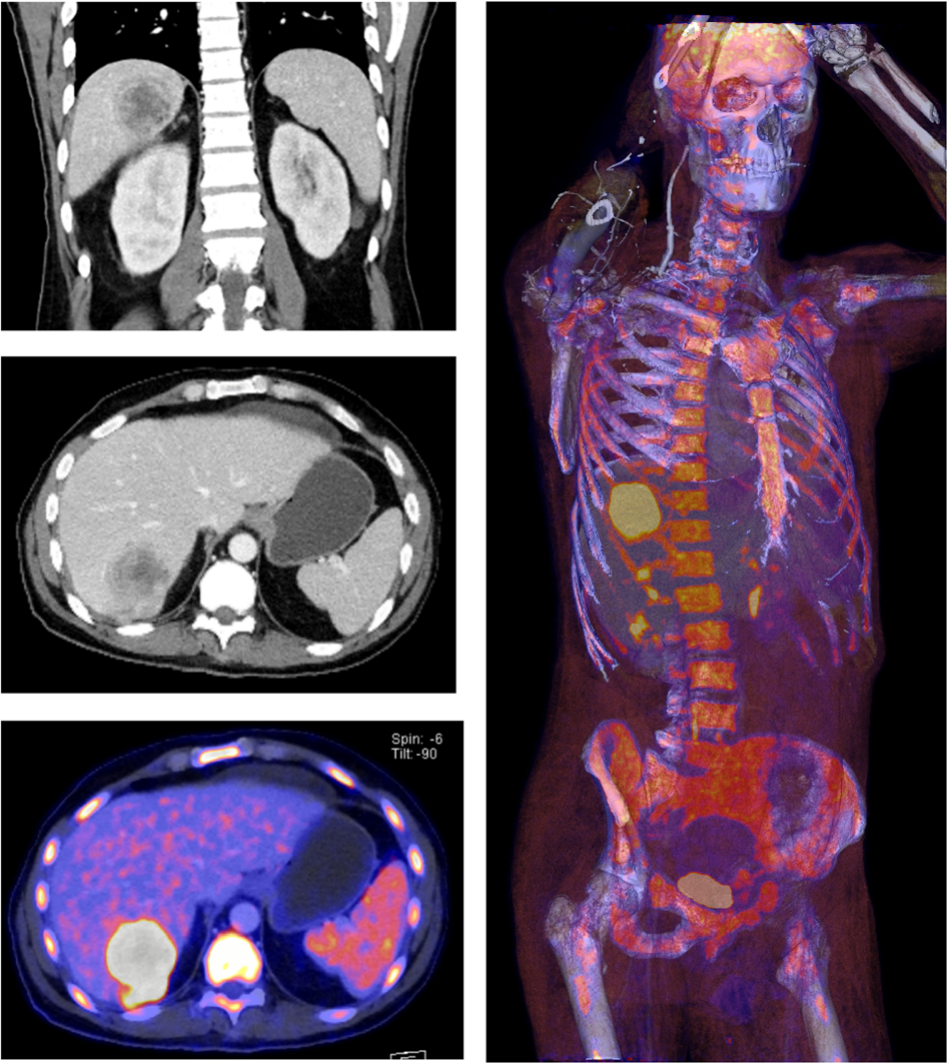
[^18^F] FDG whole-body PET-CT examination shows the known unifocal hypodense liver lesion and a distinct tracer uptake (SUV peak 33) without evidence of further suspicious lesions. Additionally, an increased signal in the entire bone marrow was found
